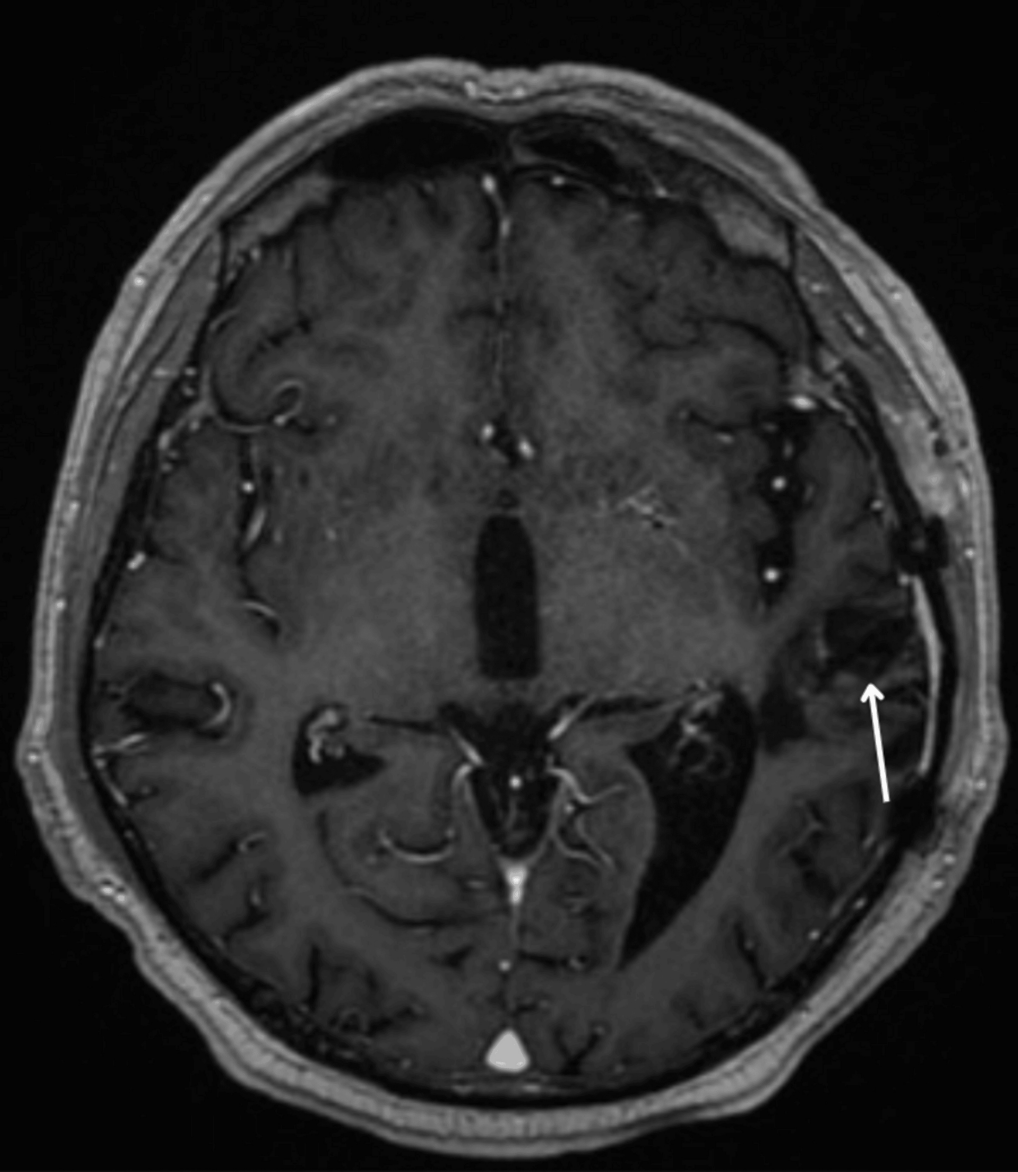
A control brain MRI was performed at three months postoperation. A cavity is shown following resection, edited with slight contrast enhancement for better visualization.
